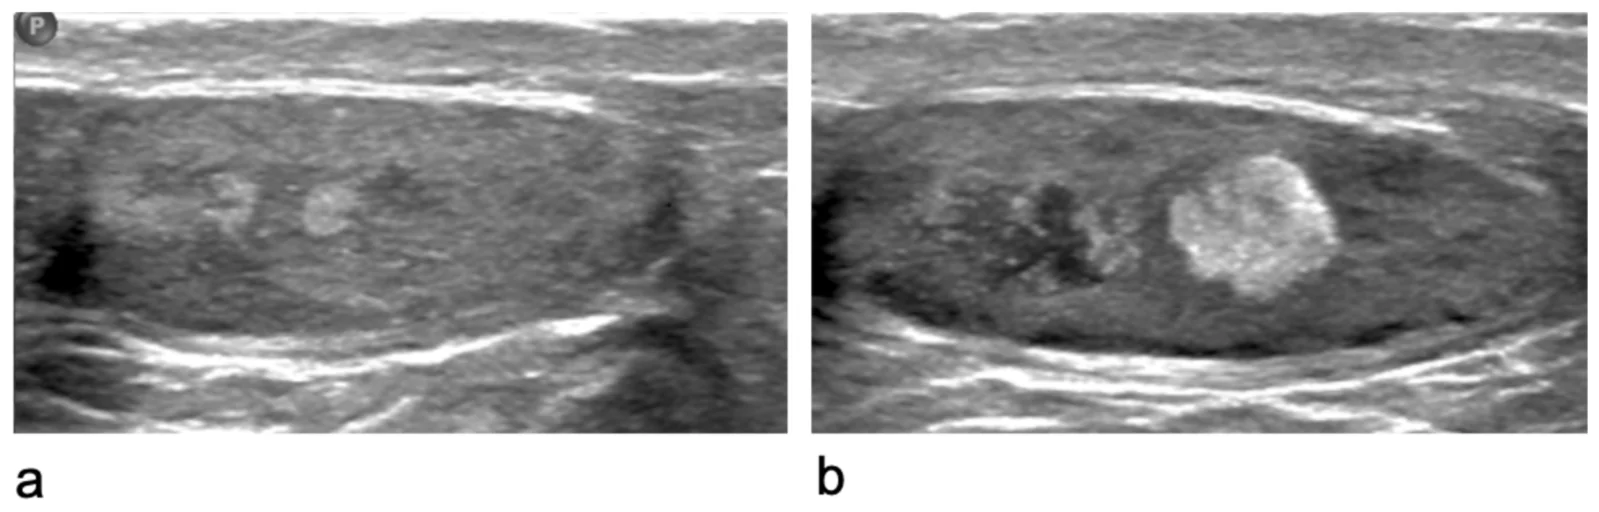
A 26-year-old man was referred for ultrasound evaluation because of azoospermia and markedly hypotrophic testes. The right (a) and left (b) testes were small, with heterogeneous echotexture and multiple non-palpable lesions of variable echogenicity. The diagnosis of Klinefelter syndrome was confirmed by karyotyping.
